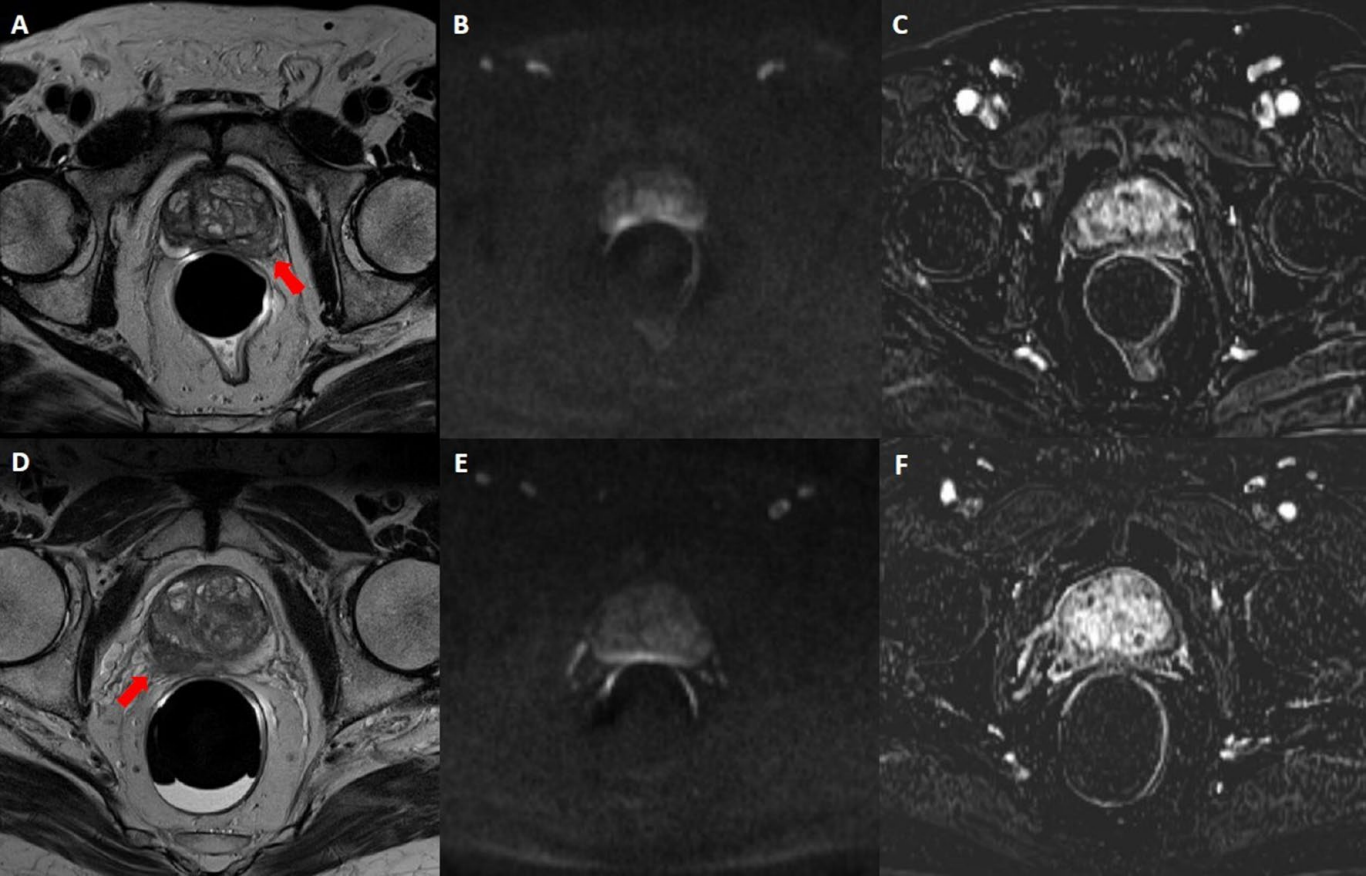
PI-RADS 3 scored alterations. In the first row, an example of PI-RADS 3 lesion confirmed at biopsy as GS 3 + 3: mpMRI showed reduced T2 signal intensity in the left PZ (A), altered diffusivity at b1000 DWI (B) without contrast enhancement (C). In the second row, an example of PI-RADS 3 lesion not confirmed at biopsy: mpMRI showed reduced T2 signal intensity in the right PZ (D), altered diffusivity at b1000 DWI (E) without contrast enhancement (F). *PI-RADS* prostate imaging-reporting and data system, *mpMRI* multiparametric MRI, *DWI* diffusion weighted MRI, *PZ* peripheral zone.
